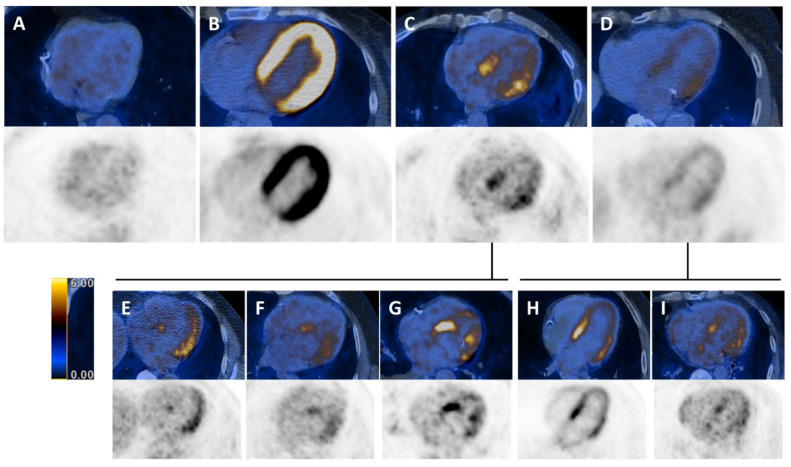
Classification of ^18^F-fluorodeoxy-glucose (^18^F-FDG) uptake patterns in the left ventricular wall. Positron emission tomography (PET) images superimposed on computed tomography (CT) images (colored) and PET images alone (black and white). (A) No myocardial ^18^F-FDG uptake. (B) Diffuse myocardial ^18^F-FDG uptake. (C) Focal myocardial ^18^F-FDG uptake. (D) Focal on diffuse myocardial ^18^F-FDG uptake. (E) Focal over half. (F) Focal spot. (G) Focal ring. (H) Focal on diffuse over half. (I) Focal on diffuse spot.
